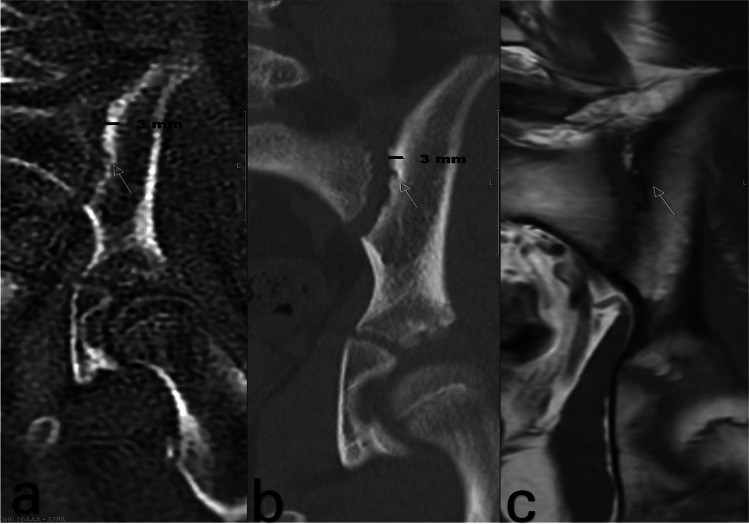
A 16-year-old boy with sacroiliitis. a Coronal ZTE-MRI shows millimetric cortical erosion in the left iliac wing (*arrow*) and increased density and signal thickness caused by subcortical sclerosis (*black straight lines*). b Coronal low-dose CT image shows millimetric cortical erosion in the left iliac wing (*arrow*) and increased density and signal thickness caused by subcortical sclerosis (*black straight lines*). c Coronal T1-weighted image shows millimetric cortical erosion in the left iliac wing (*arrow*) and subcortical sclerosis are not distinguishable. *CT*, computed tomography; *ZTE-MRI*, zero–time echo magnetic resonance imaging
